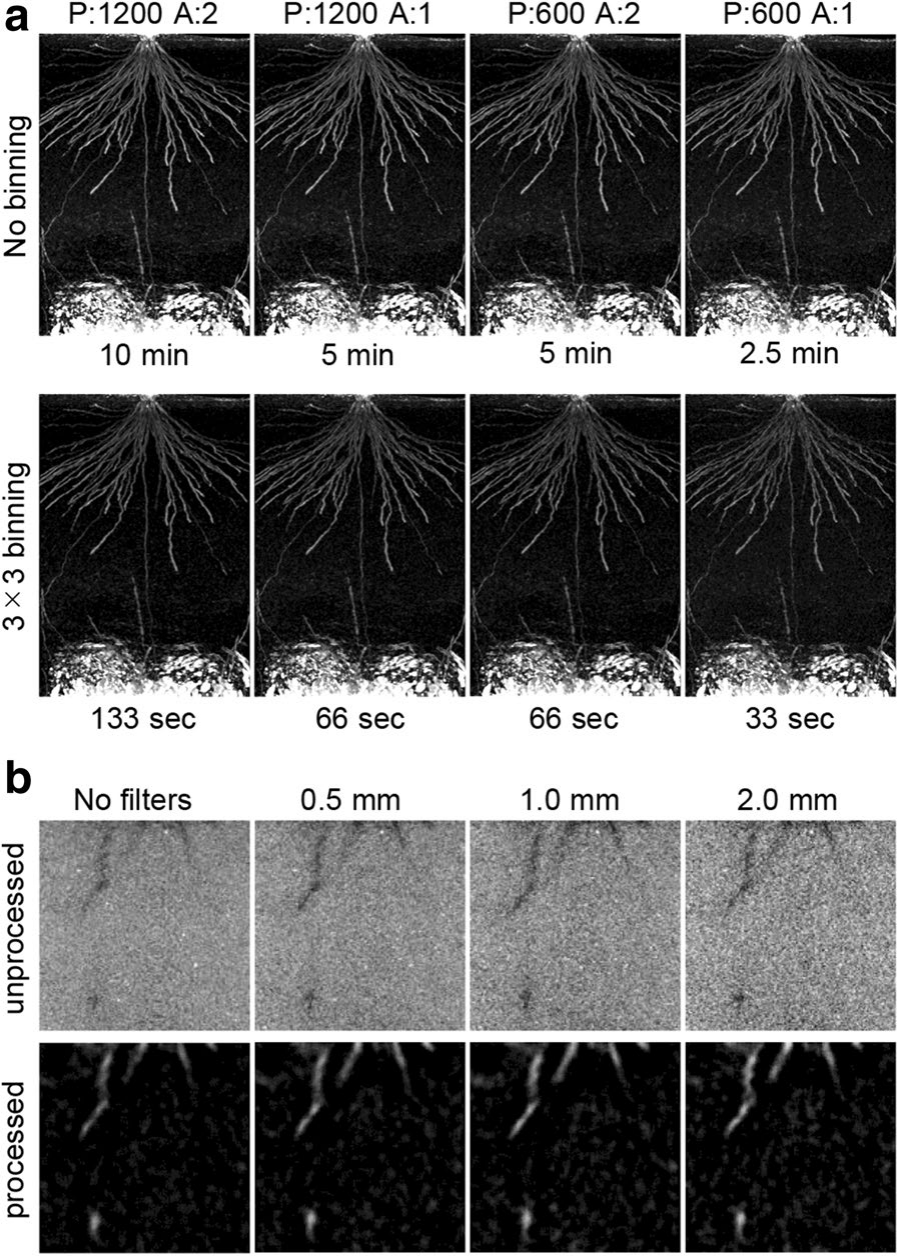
Scanning conditions to reduce X-ray dose: a various conditions for rapid CT scanning. An 8-week-old Kinandang Patong was scanned, using X-ray CT scanning with different scanning parameters. Horizontal projections were computed by the image-processing algorithm, and scanning times are shown below the images. P = number of projections, A = number used for signal averaging; b influence of Cu filters on the X-ray CT images. A pot with a 4-week-old Kinandang Patong was scanned using X-ray CT, without and with 0.5, 1.0, and 2.0 mm Cu filters. Scaled-up vertical slices of unprocessed and processed CT volumes are shown
